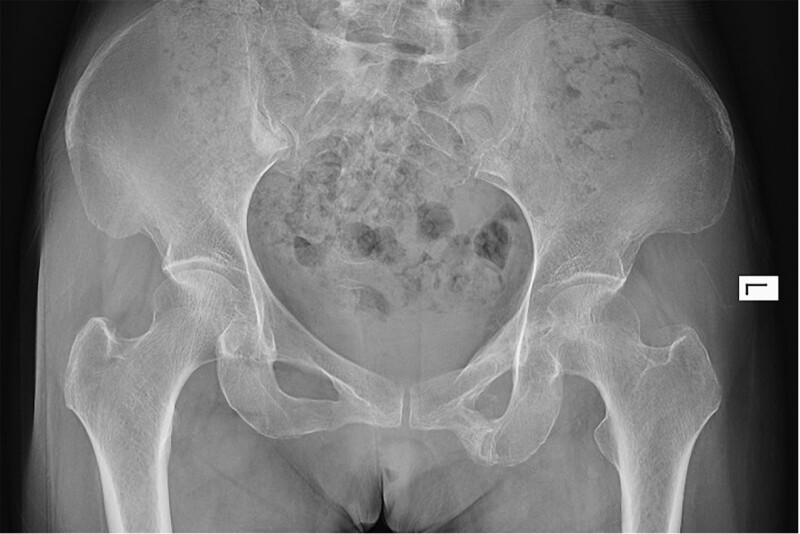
A radiograph of the pelvis taken 12 mo postoperatively reveals no recurrence or new ossified lesion formation.
